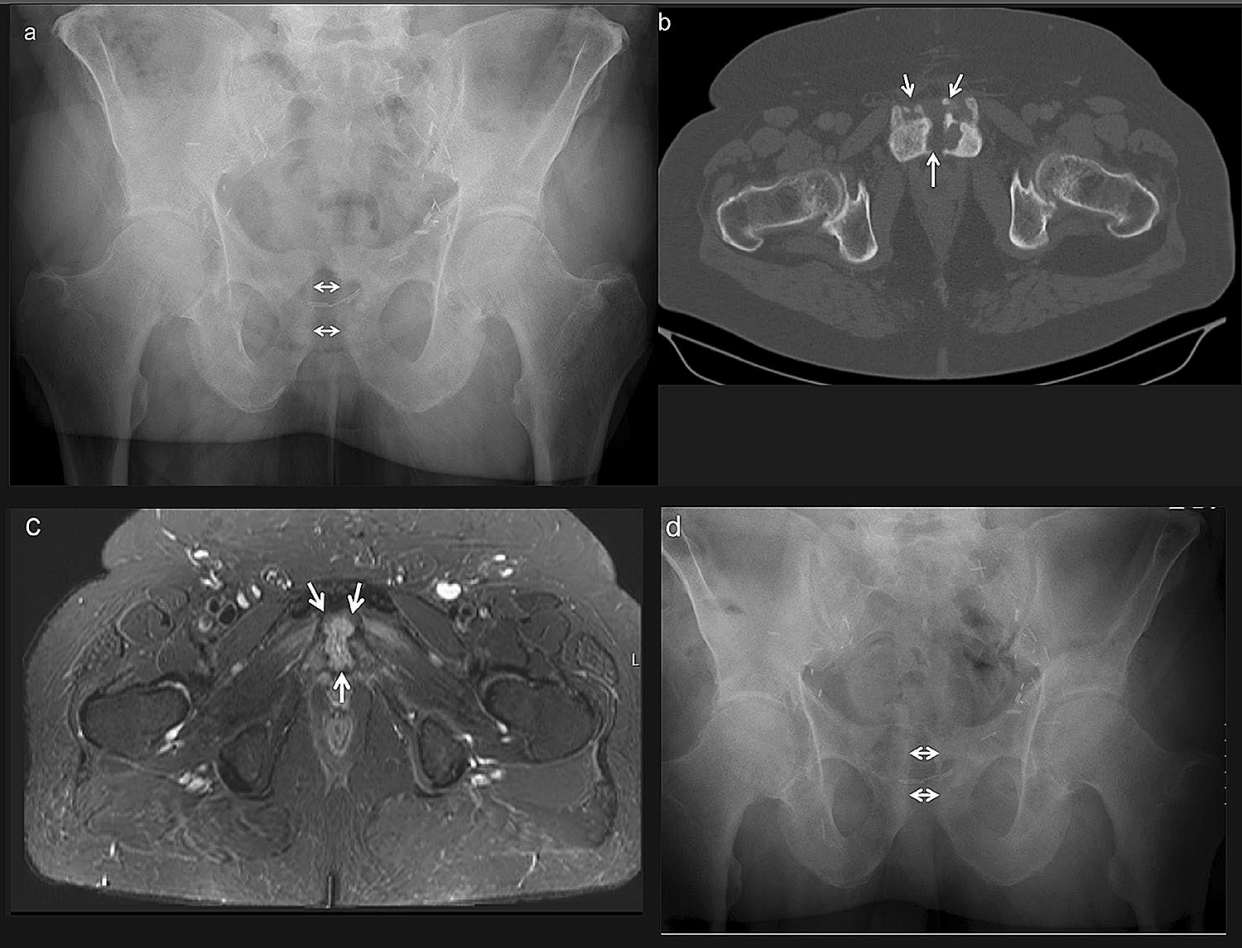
a–d A 69-year-old male with infected pubic symphysitis. Preoperative anteroposterior radiograph of the pelvis showing a slight widening of the pubic symphysis (arrows). Surgical clips of the previous prostatectomy are visible on both sides (a). CT slice of the anterior pelvis shows irregularities of the bony surfaces and necrotic bone fragments in the pubic symphysis joint space (arrows) (b). T2-weighted MRI transection of the pubic symphysis shows a fluid collection in and behind the joint space (arrows) (c). Postoperative anteroposterior radiograph of the pelvis with similar widening of the pubic symphysis as preoperative (arrows) (d)
